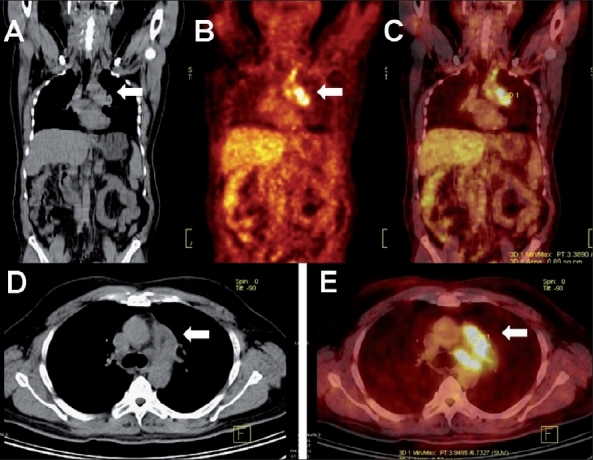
Mediastinal lymphadenopathy in a patient of carcinoma colon who, post-hemicolectomy and post-chemotherapy was detected to have raised tumor marker (CEA) levels. Coronal plain CT (A), PET (B), PET/CT (C) with axial plain CT (D), and PET/CT (E) images of the thorax show FDG-avid mediastinal nodes (SUV 6.7) (arrows). No pulmonary lesion was detected on the CT images. The patient underwent mediastinoscopy and lymph node biopsy, which revealed tuberculosis
